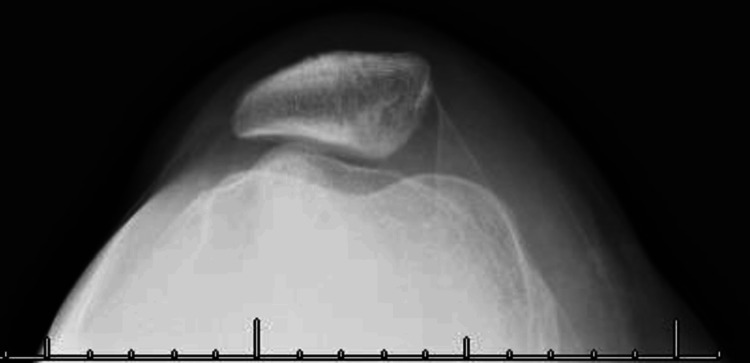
Post-operative axial X-ray of patellofemoral articulation portraying removal of osteochondral defect from inferior aspect of medial patellar facet.
